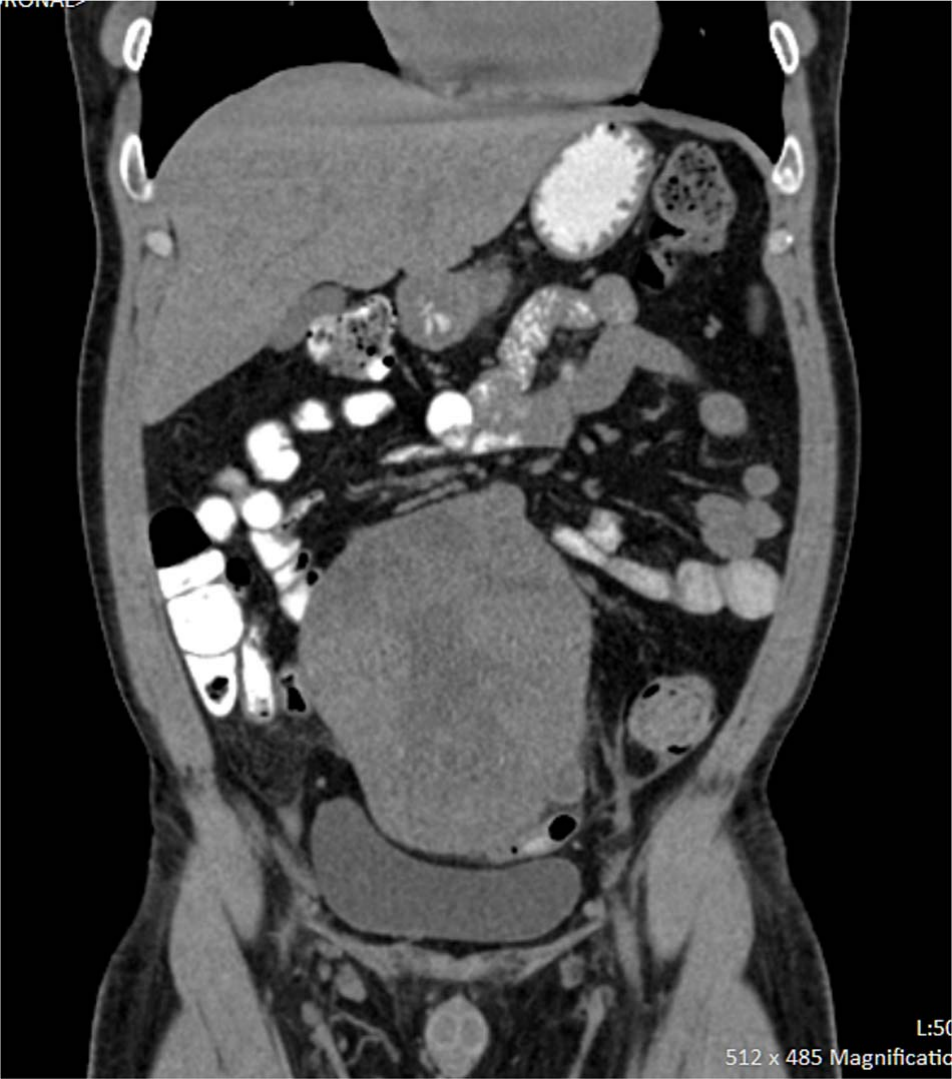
CT scan showing a lobulated enhancing mass arising from the rectosigmoid region with focal necrosis.
